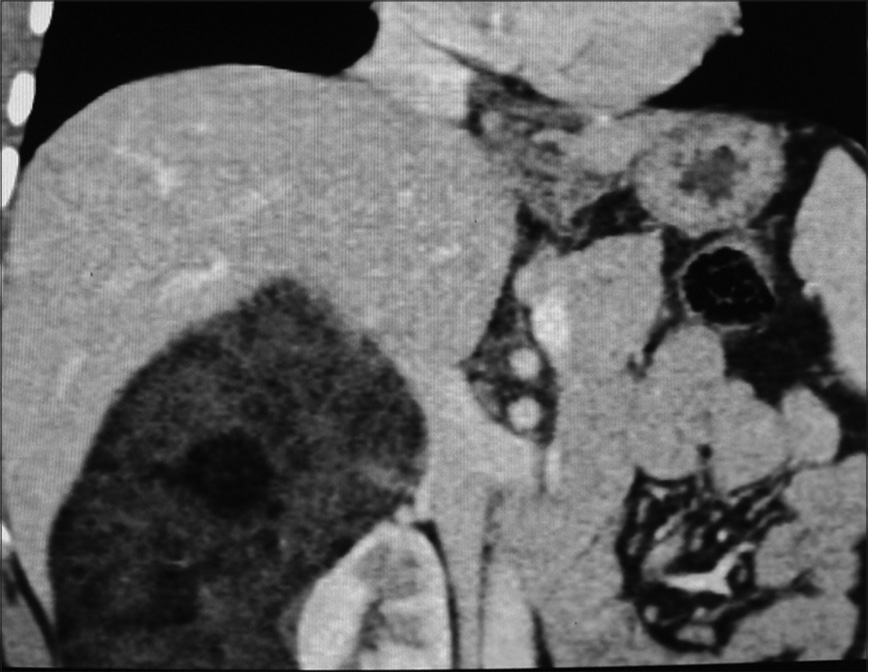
Coronal reformation contrast enhanced CT shows the fatty mass with some dense components on the superior and lateral aspects of right kidney displacing it medially
